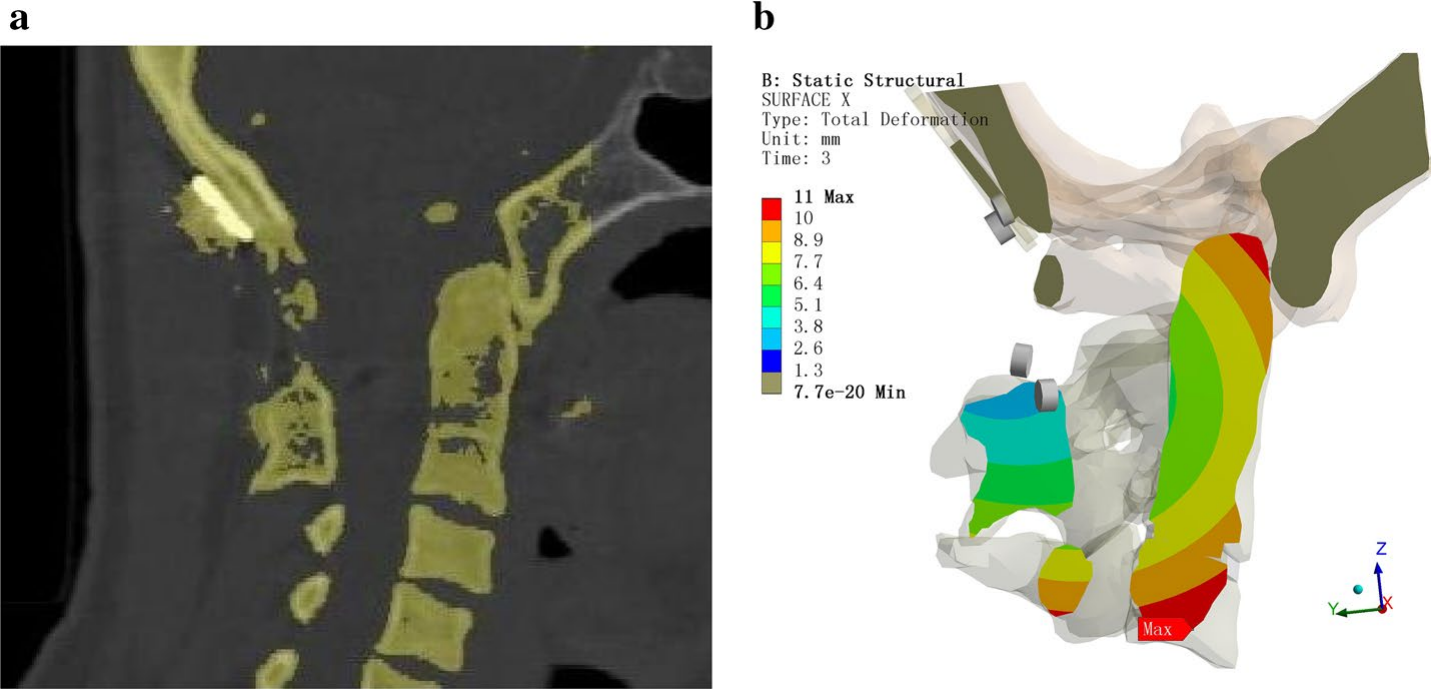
Comparison chart for the mid-sagittal plane. a Computed tomography (CT). b ANSYS displacement cloud chart
